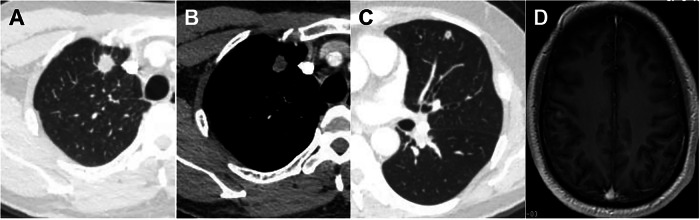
Images in a 50-year-old man with distant metastasis after surgical resection of acinar-predominant adenocarcinoma in clinical stage I. A, B Axial contrast-enhanced CT image with a lung window setting shows a 15 mm spiculated pure-solid nodule in the right upper lobe. The preoperative clinical staging of the patient was T1bN0M0, with an overall stage of IA2. The lesion was pathologically confirmed as acinar-predominant adenocarcinoma without lymph node metastasis and lymphatic and vascular involvement (LVI), but with VPI. Therefore, the postoperative pathological staging of the patient was T2aN0M0, with an overall stage of IB. Genetic evaluation indicated that the lesion carried an *EGFR* mutation. C, D Two years after the surgery, postoperative examination revealed distant metastases, including multiple pulmonary pure-solid nodules, rib metastases, and brain metastasis. Cancer-specific death had occurred after 68 months of follow-up
